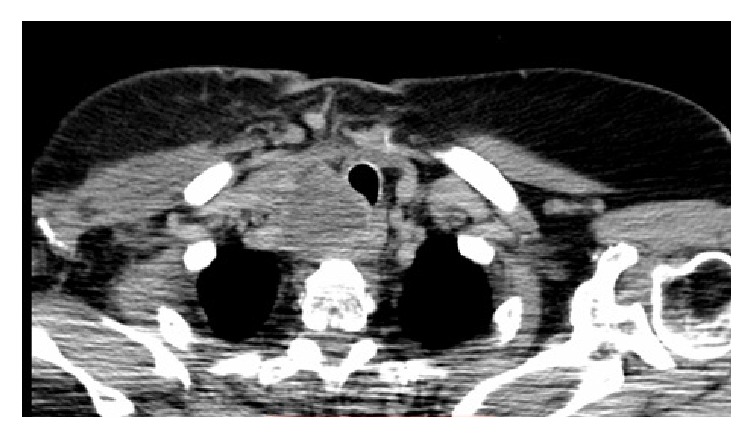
Axial chest CT showing a hypodense, solid, infrahyoid mass extending to the superior mediastinum.
